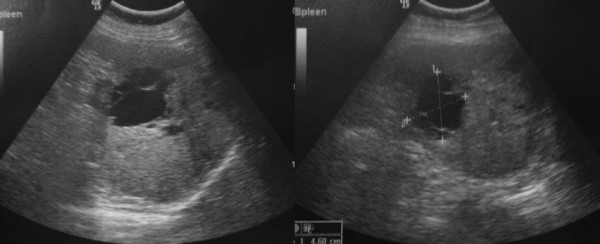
Ultrasound of the spleen demonstrates enlarged spleen with well-defined hypoechoic, and hyperechoic lesions.
